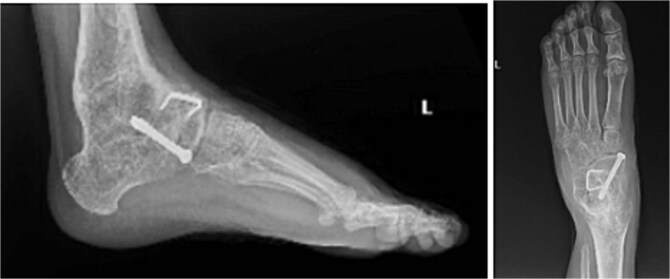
Radiographs of the left foot demonstrating progressive talonavicular joint degeneration and dorsal exostosis formation. Lateral and dorsoplantar views show degenerative changes and bony overgrowth contributing to midfoot pain and mechanical symptoms prior to subsequent surgical intervention.
